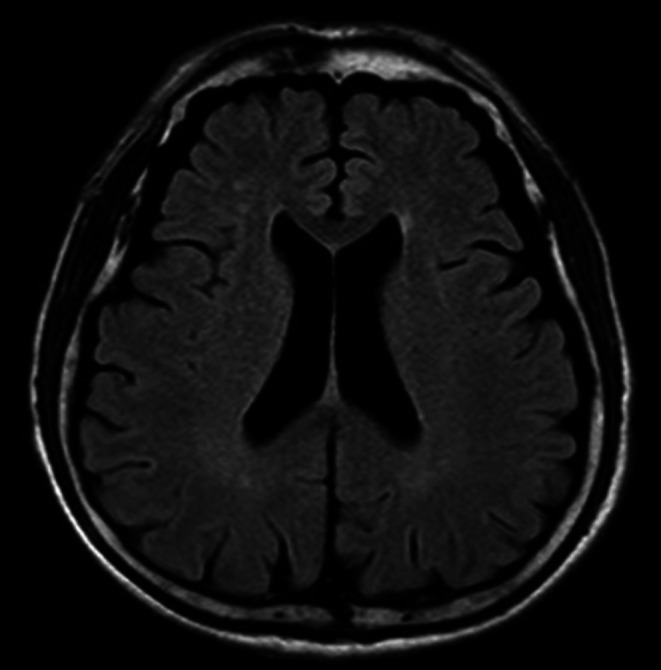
Magnetic resonance imaging. No definite areas of abnormal high signal intensity are identified on diffusion‐weighted imaging. There are no high signal lesions on T2‐weighted or FLAIR images suggestive of encephalitis. No obvious intracranial hemorrhage or gross cerebral infarction is observed.
